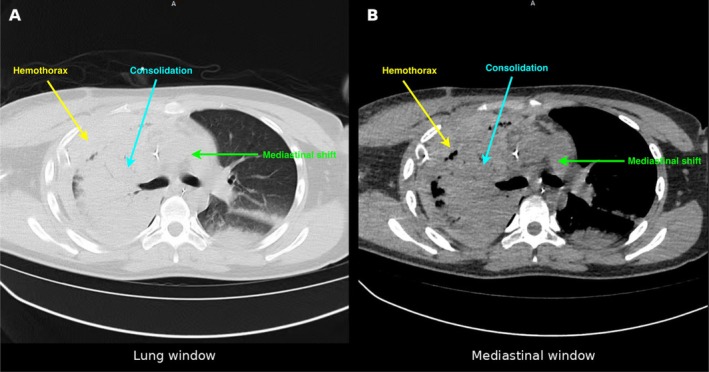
Chest CT on Day 5 (January 21, 2025). (A) Lung window showing massive right hemothorax with lung consolidation and mediastinal shift to the left. (B) Mediastinal window demonstrating the extent of pleural hematoma and hepatic compression. Yellow arrows indicate hemothorax; cyan arrows indicate consolidation; green arrows indicate mediastinal shift.
